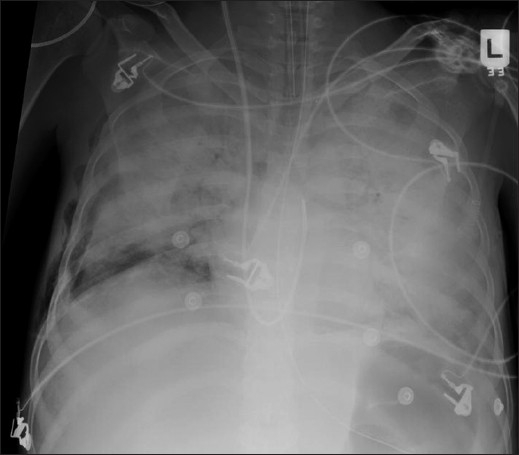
A patient's AP chest radiograph showing worsening of the air space shadowing with a further complication of a right-sided pneumothorax
